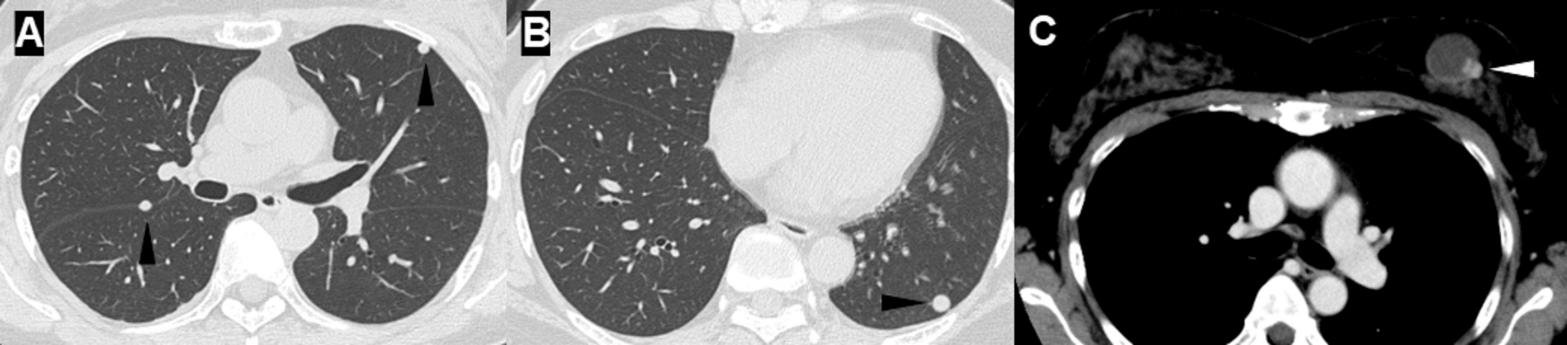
Chest and breast imaging findings on computed tomography Chest computed tomography showing multiple, well-defined nodules in both lungs (A, B; arrowheads) and a cystic lesion with a solid component in the left breast (C; arrowhead)
